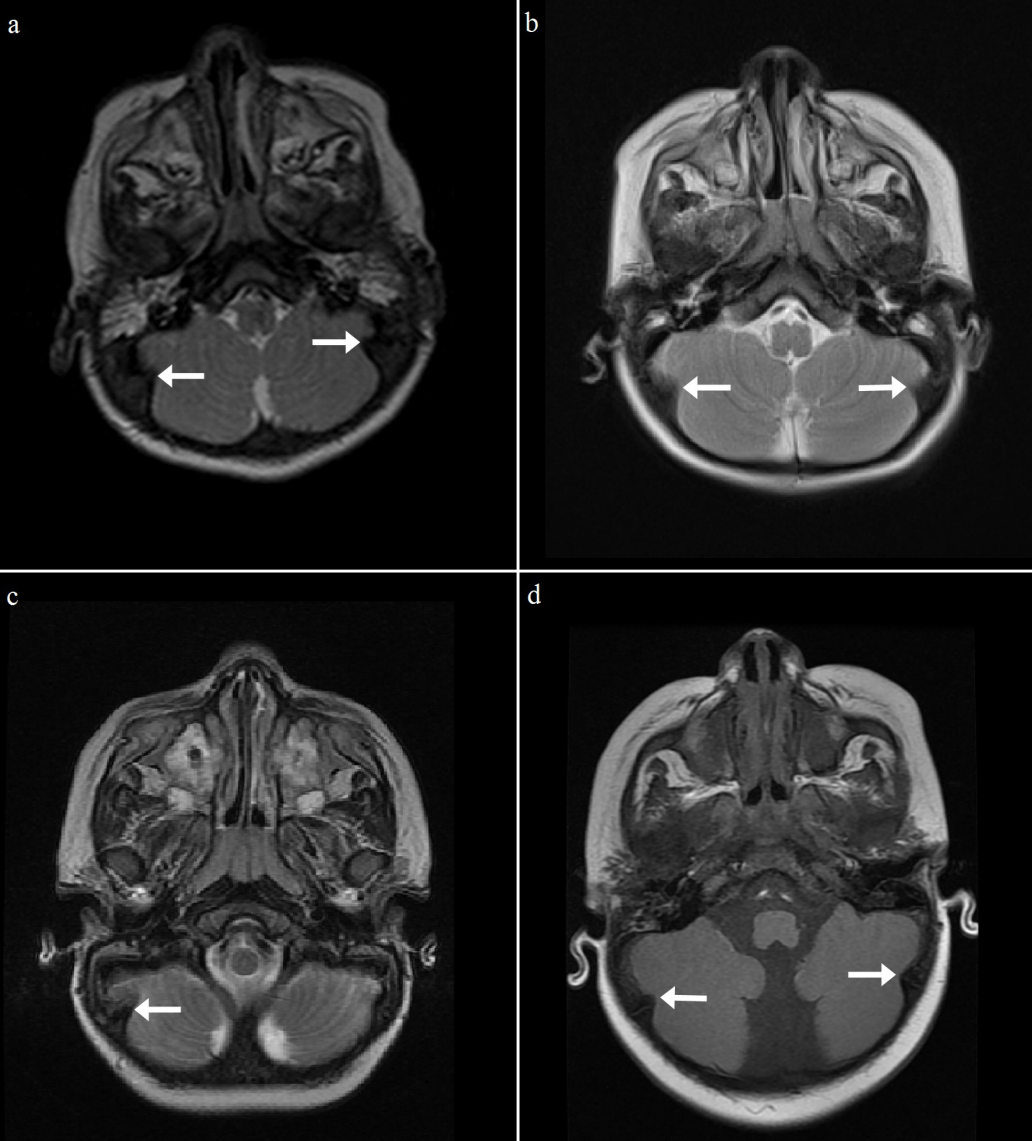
Axial T2 weighted (a–c) and T1 weighted (d) MR images show unilateral or bilateral IHOMs (white arrows) of 4 different patients with MPS type II. (a: 14 months old, b: 15 months old, c: 4 years old, d: 8 months old).
